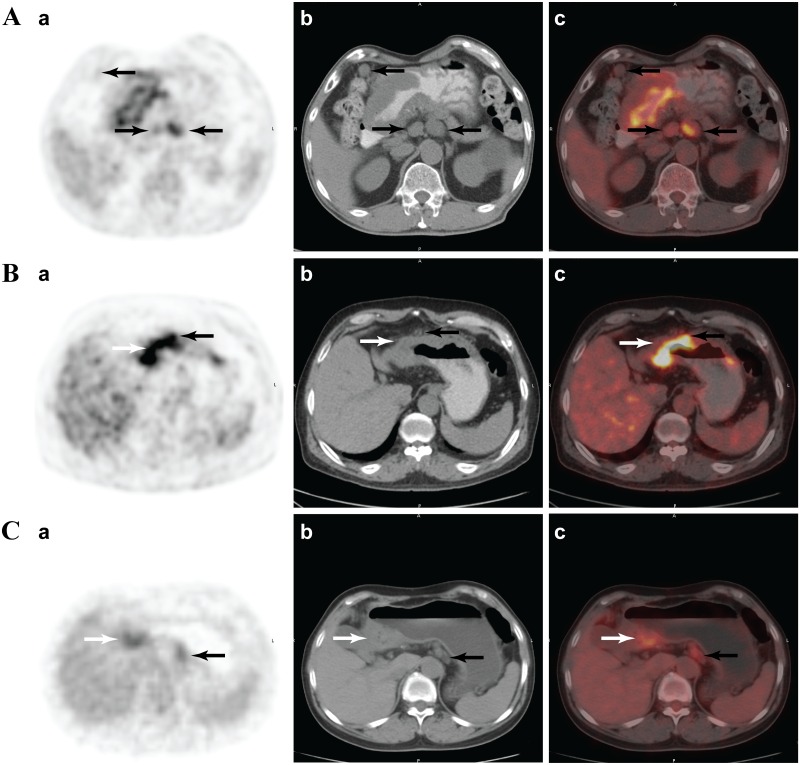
Analyses of regional lymph nodes. (A) A 57-year-old male with mucinous adenocarcinoma in gastric antral (white arrow) and metastatic lymph nodes (30/37). Three solitary lymph nodes with fluorodeoxyglucose (^18^F-FDG) uptake higher than surrounding fat tissue were evaluated as MPLN (black arrows). (B) A 62-year-old male with moderately to poorly differentiated adenocarcinoma in gastric antral and metastatic LN (3/17). A prominent nodular ^18^F-FDG uptake spot (black arrow) was observed in the stomach wall of the primary lesion (white arrow). The same level CT cross-section image showed a soft nodule adhesion in the gastric wall, and was counted as a MPLN. (C) A 62-year-old women with poorly differentiated adenocarcinoma in gastric antral and metastatic LN (34/52). An ^18^F-FDG uptake spot (black arrow) was noted in the rear gastric body and identified as a LN cluster. The cluster was counted as three MPLNs (a. Positron emission tomography imaging; b. the same slice with computed tomography imaging; c. positron-emission tomography and computed tomography fusion imaging).
